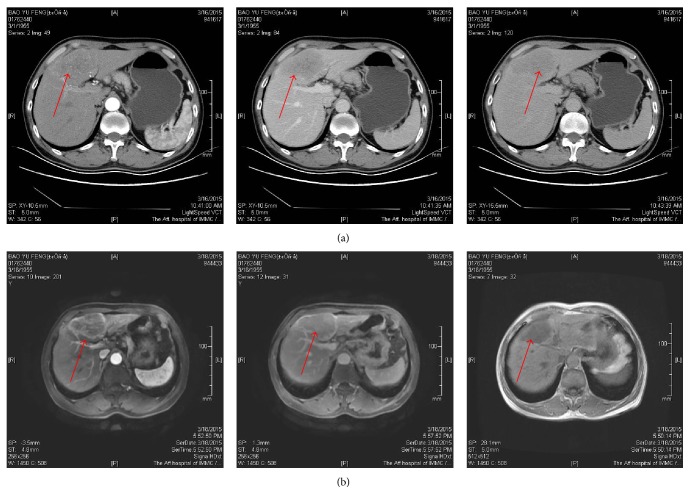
A 60-year-old man has a nodule of low density in the left hepatic lobe segment. (a) Multiphase CECT showed significant enhancement in arterial phase, the enhancement extent fade in portal and delay phase. (b) Multiphase contrast enhanced MRI showed typical enhancement pattern (red arrows in a, b). In conclusion, the lesion in the left hepatic lobe was consistent with PHC.
